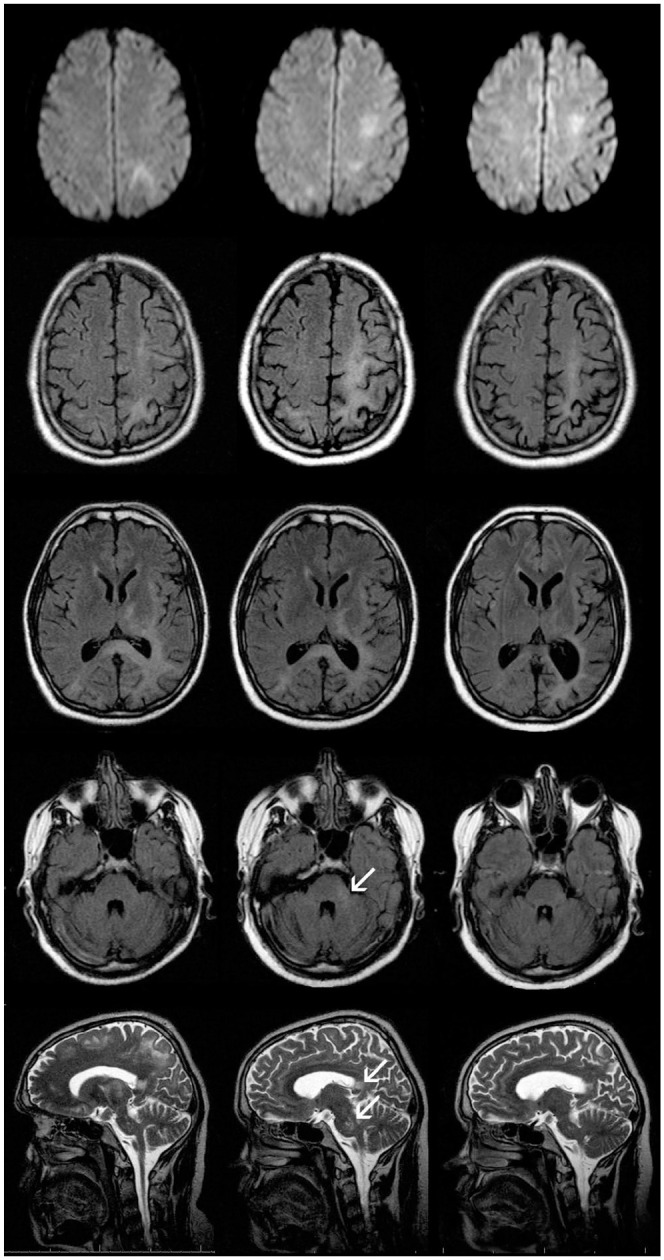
Axial DWI (upper row), T2-FLAIR (three middle rows) and sagittal T2-TSE (lower row) in patient 1. Left column shows images performed approximately 3 months after onset of the neurological symptoms (right sided homonymous hemianopsia and ataxia) and showing T2-hyperintense confluent subcortical lesions with left parieto-occipital focus but also involving left motoric and premotoric cortex, left parietal and occipital cortex, and subcortical white matter, posterior internal capsule, external capsule, and splenium of corpus callosum. In these areas, patchy diffusion restriction but no gadolinium enhancement is observed. The follow-up after 2.5 months (middle column) indicates progression of the subcortical PML lesions with left hemispheric accentuation (e.g. left cella media) but also new lesions in left pons and cerebellar pedunculus. Arrows indicate lesions in cerebellar pedunculus, splenium of corpus callosum, and pons. Approximately 6 months later (right column), decrease of the PML lesions with increasing atrophy and consecutive *e vacuo* enlargement of the inner CSF spaces can be noticed. Moreover, the infratentorial PML lesions are barely visible. CSF, cerebrospinal fluid; DWI, diffusion weighted imaging; PML, progressive multifocal leukoencephalopathy.
